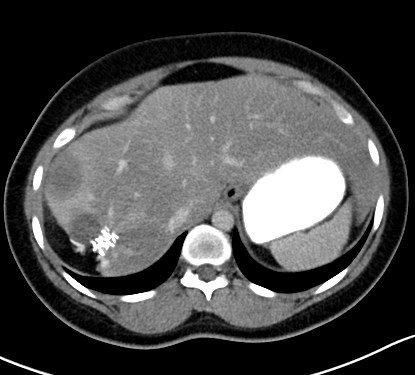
Repeat abdominal CT after initial hepatic resection. Several new, low-attenuation lesions are present in the right hepatic lobe. Similar, smaller lesions were present in the left hepatic lobe (not shown).
